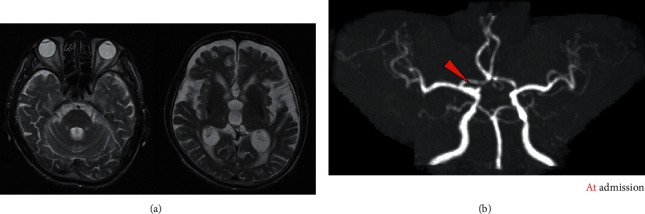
On admission: brain MRI showing no abnormalities in the cerebraand parenchyma on T2-weighted images (a). MRI shows a 3 mm aneurysm in the siphon of the internal carotid (arrow head) (b).
